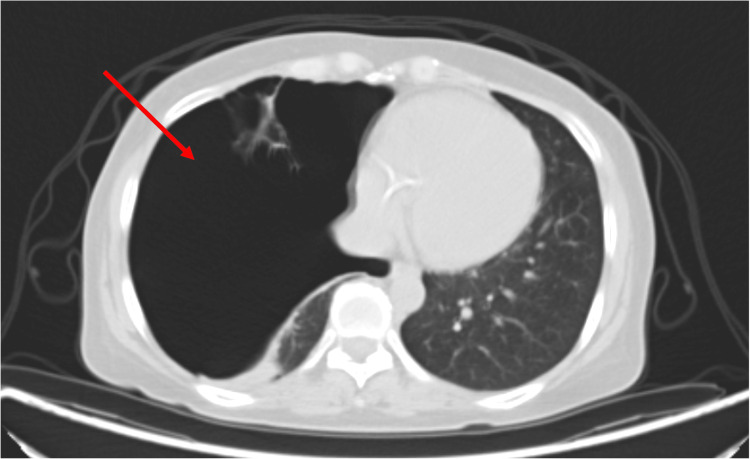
PET/CT scan showing a large right-sided pneumothorax (arrow) with near complete atelectasis of the remaining right hemithorax.
